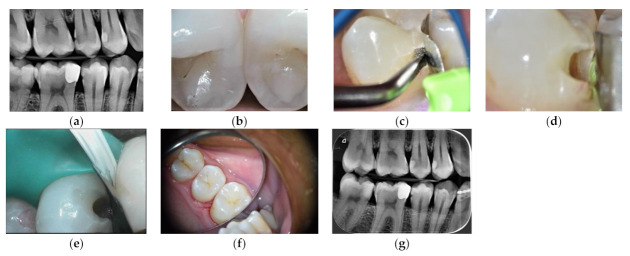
Slot cavity preparation on a distal carious lesion of an upper premolar restored with HV-GIC LC: (a) pre-operative radiograph; (b) marginal crest modification white spot (blue arrow); (c) US diamond insert half-round shape; (d) slot preparation; (e) metal matrix setting; (f) final occlusal view; (g) post-operative radiograph.
